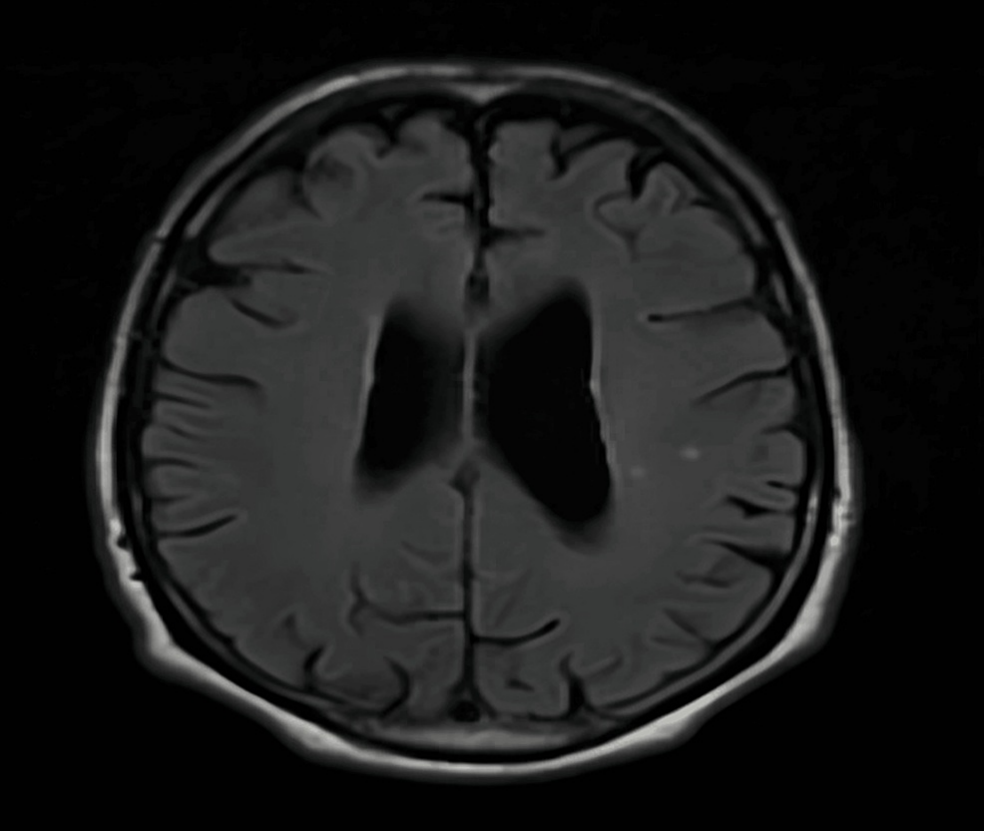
Brain MRI axial imaging without any remarkable results.
